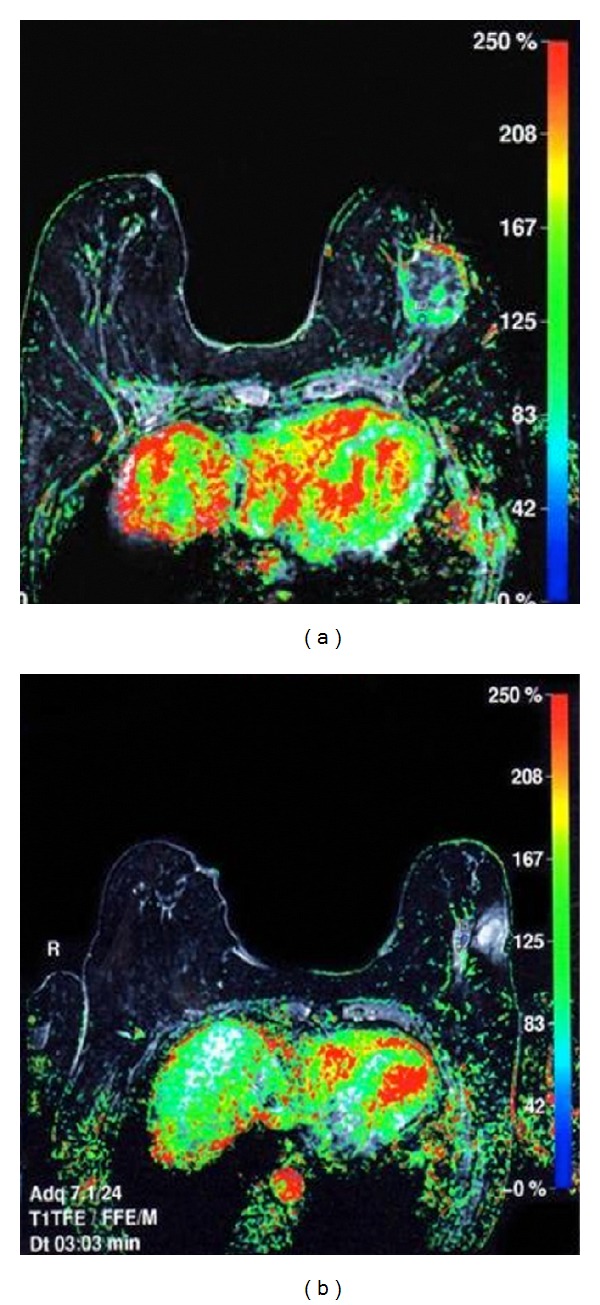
MRI during neoadjuvant treatment monitoring, where one can visualize macroscopic tumor disappearance corresponding to greater partial response.
